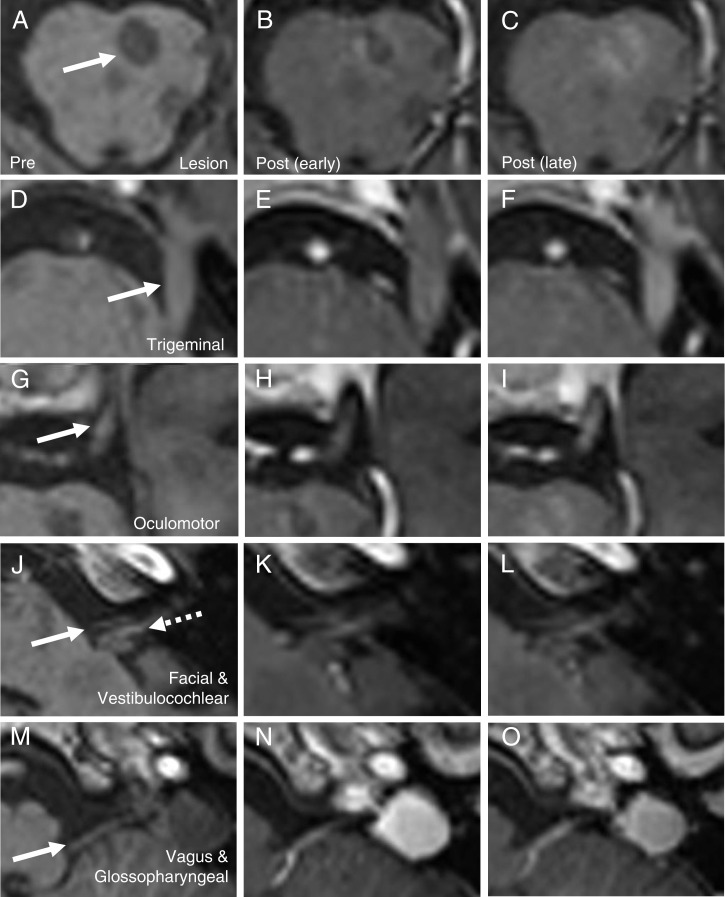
The evolution of contrast enhancement on T1-weighted images at early (7 min) and late (49 min) stages, for the pontine lesion (*A–C*), and ipsilateral trigeminal (*D–F*), oculomotor (*G–I*), facial and vestibulocochlear (*J–L*), and vagus and glossopharyngeal nerves (*M–O*). Arrows indicate the pontine lesion (*A*), trigeminal nerve (*D*), oculomotor nerve (*G*), facial nerve (*J*, solid arrow), vestibulocochlear nerve (*J*, dotted arrow), and the vagus/glossopharyngeal nerves (*M*).
